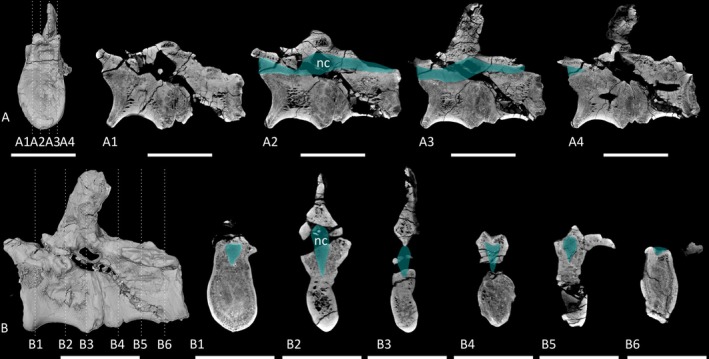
CT scan of *Pampadromaeus barberenai* anterior caudal vertebrae ULBRA‐PVT016. (A, B) 3D rendering (A) anterior view, (B) lateral view; (A1–A4) CT scan slices in parasagittal cross‐section, (B1–B6) CT scan slices in transverse cross‐section. nc, neural canal. Key: Blue highlight, neural canal. Scale bars = 20 mm.
